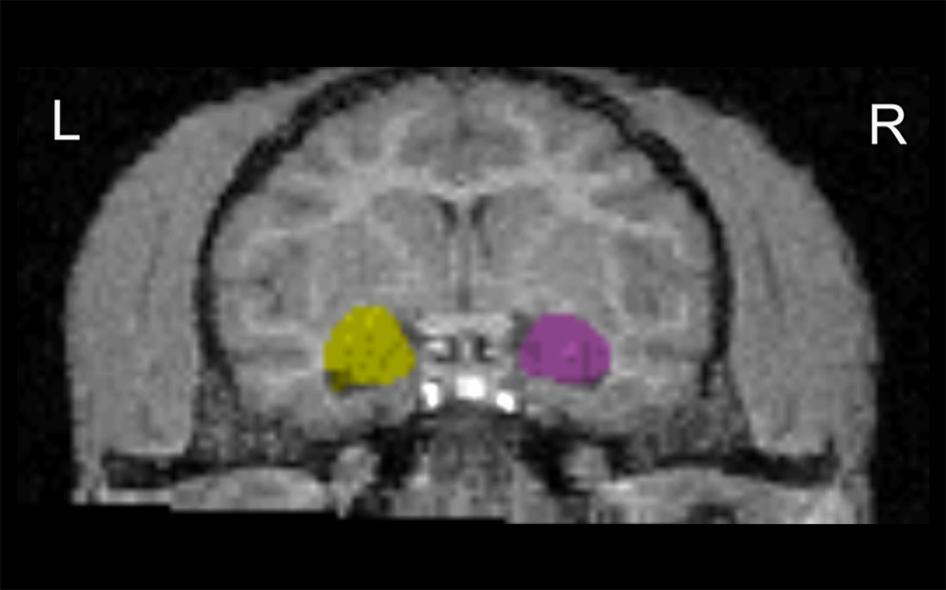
Coronal view of the amygdala outline in T1-weighted images. Images were aligned to the hippocampus axis. L, left hemisphere; R, right hemisphere.
